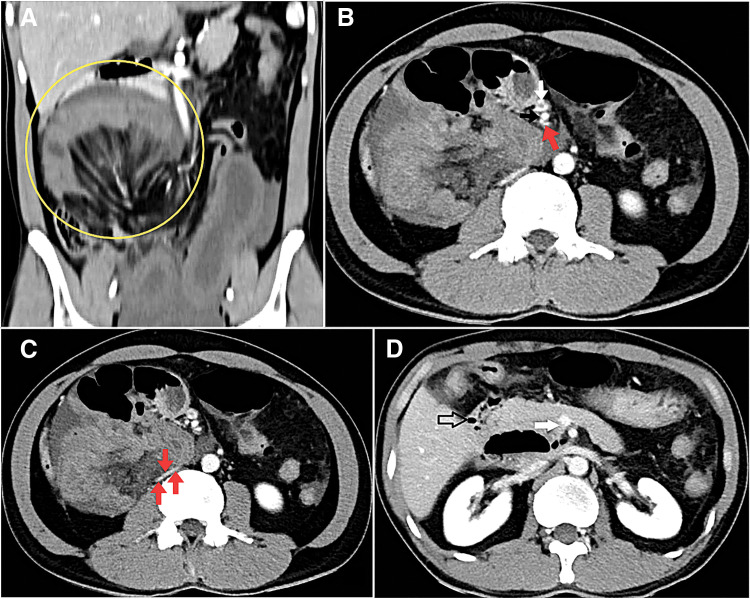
CT scan on admission. (A) On coronal images, the small intestine is displaced to an abnormal position near the right abdominal wall (yellow circle), with the right colon absent from its normal anatomical location. (B) Axial images showed the jejunal artery and vein branches (red arrow) wrapped from the front, around the SMA (black arrow), to its posterior right (SMV–white arrow). (C) The inferior vena cava (red arrows) was compressed by a hernia sac. (D) The 3rd part of the duodenum (open arrow) did not run to the left side, and the SMV (white arrow) is on the right side of the SMA. SMA, superior mesenteric artery; SMV, superior mesenteric vein
